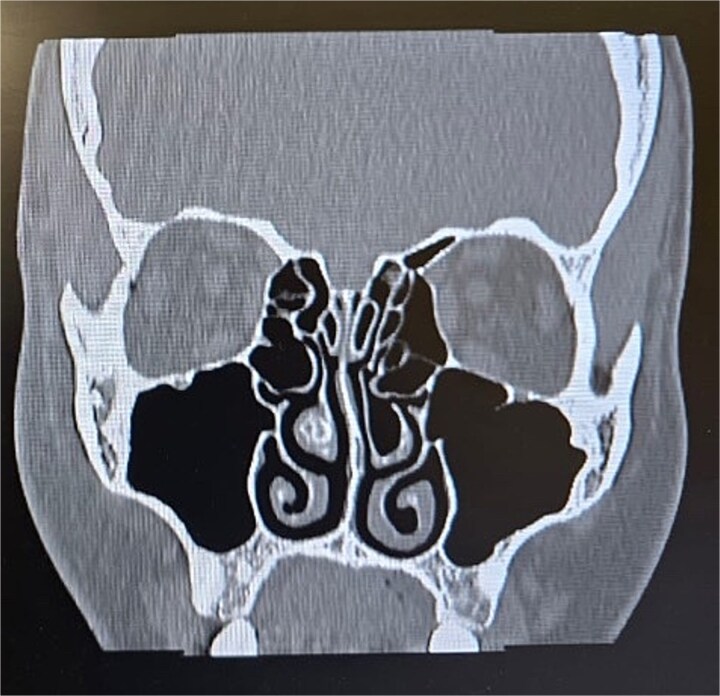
Paranasal sinus CT scan in the coronal plane demonstrating left middle concha bullosa and inferior concha bullosa.
